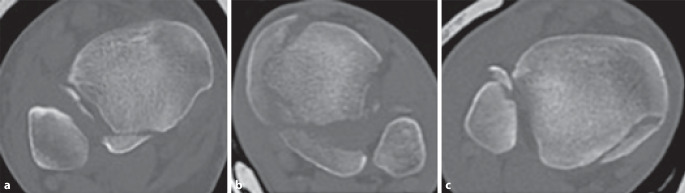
Primär wurden für alle Patienten die präoperativen Röntgenaufnahmen des OSG im anteroposterioren (a.-p.), bei 20°-Innenrotation („Mortise“-Aufnahme) sowie im lateralen Strahlengang entsprechend dem Unfallmechanismus nach Lauge-Hansen klassifiziert. Haraguchi et al. beschrieben 44 Frakturen vom Supinations-Eversions-Typ, 7 Frakturen vom Pronations-Eversions-Typ sowie 6 Verletzungen vom Pronations-Abduktions-Typ. Die Auswertung der CT (2–3 mm Schichtdicke) erfolgte axial auf Höhe der tibialen Gelenkfläche. Gemessen wurde die anteilige Frakturgröße des posterioren Malleolus im Verhältnis zur gesamten Gelenkfläche. Zudem bestimmten sie den Winkel zwischen der Malleolengabel und der Hauptfrakturlinie.
